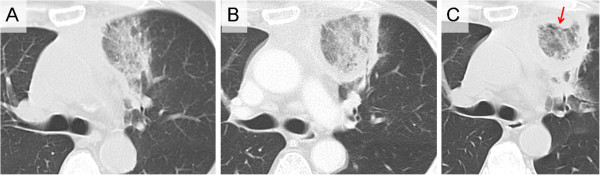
Sequential chest computed tomography images in patient with invasive pulmonary mucormycosis presenting reversed halo sigh. (A) On 39th (−39th) day before the operation, chest computed tomography (CT) revealed ground-glass opacity (GGO). (B) On -28th day, chest CT revealed reversed halo sign (RHS) comprising both central GGO and the outer rim recognized as a ring-shaped high-density area replacing the outside parenchyma of GGO. (C) On -11st day, chest CT revealed RHS air crescent-like small air slit (the tip of an arrow) at the junctional area between GGO and the outer rim.
